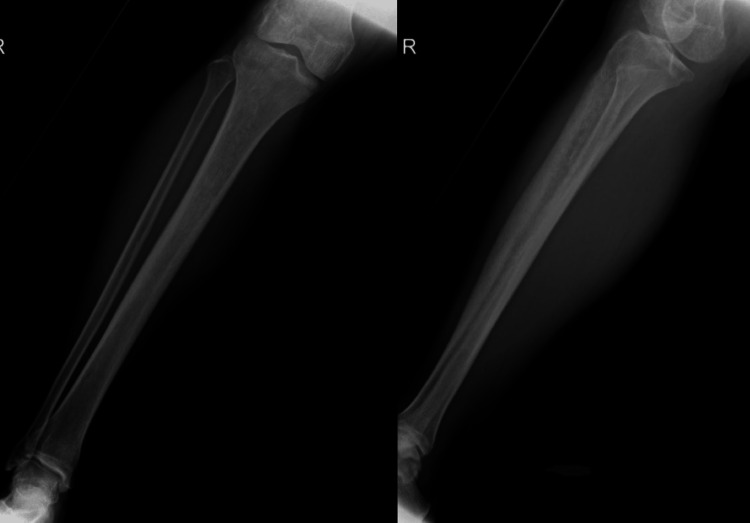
X-ray: AP and lateral views, three months after surgery X-ray showed ill defined, intramedullary, mixed sclero-lytic lesion in meta-diaphysis and proximal tibia with cortical thickening and wide zone of transition. There was no periosteal reaction, joint involvement, or soft tissue enhancement. The sclerotic areas were attributed to calcium phosphate bone cement inserted during surgery.
